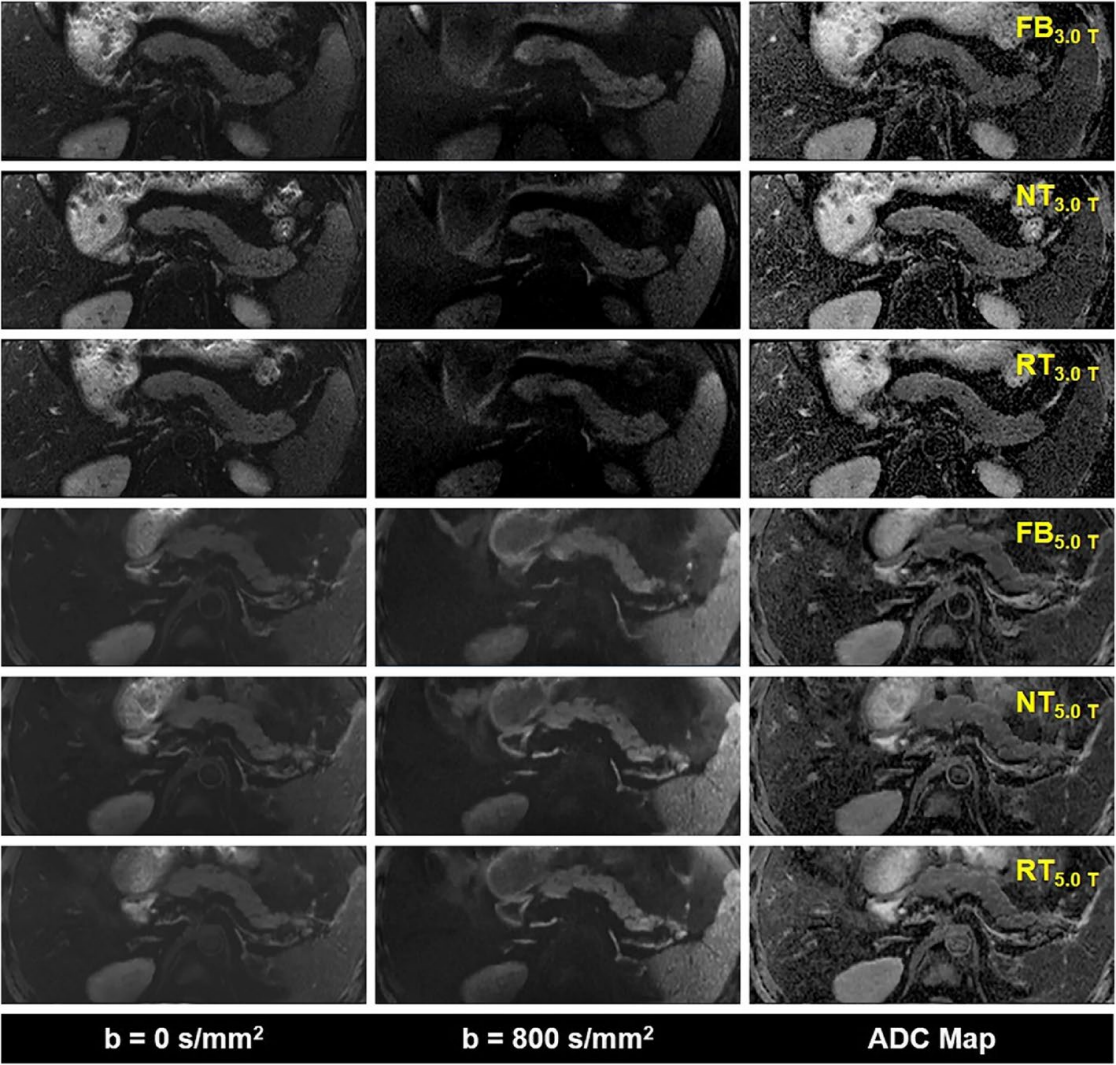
Representative MR images of a 36-year-old man weighing 75.4 kg and with a BMI of 24.9. The DWI images obtained by six acquisition approaches are listed in different rows. The DWI images of *b* = 0 s/mm^2^, *b* = 800 s/mm^2^, and ADC parametric maps are displayed in different columns
